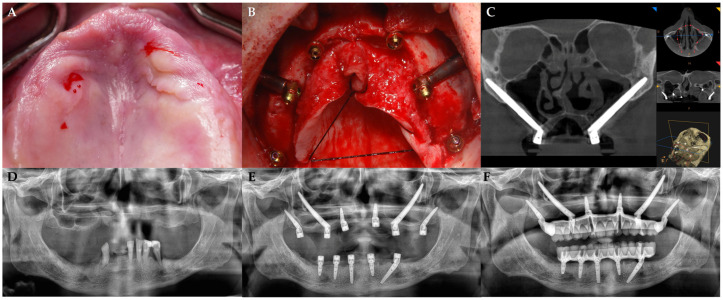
(A) Preoperative clinical view. (B) Intraoperative view after flap elevation and placement of implants. (C) Postoperative CT scans with 3D reconstruction showing the trajectory and position of zygomatic implants with A—Anterior; P—Posterior; R—Right; L—Left; H—Head; F—Foot. (D) Preoperative orthopantomogram. (E,F) Postoperative orthopantomograms showing the implants in place (E) and the final prosthetic rehabilitation (F). The abutments were attached following flap closure with non-resorbable sutures (4/0). The following post-surgical instructions were given: (1) ibuprofen 400 mg (or paracetamol 1 g for patients allergic to NSAIDs) to be taken 2–4 times a day, during meals if needed; (2) 0.2% chlorhexidine mouthwash for 1 min twice a day for 2 weeks. A soft diet was recommended for 3 weeks.
